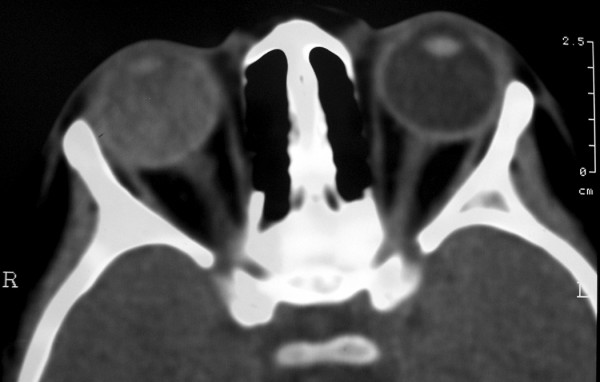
Computed Tomography. Total exsudative retinal detachment in the right eye.
